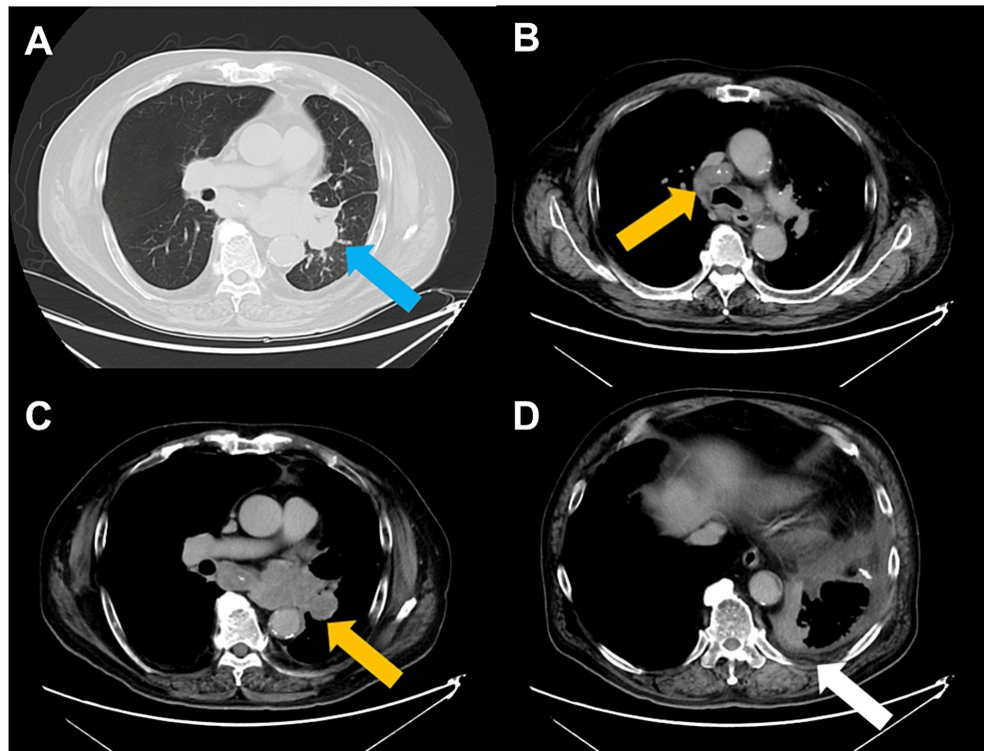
Chest computed tomography before administration of immune checkpoint inhibitor. Chest computed tomography demonstrated (A) a solitary lesion in the lower lobe of the left lung (blue arrow), (B and C) swelling of multiple hilar and mediastinal lymph nodes (yellow arrow), and (D) pleural dissemination (white arrow).
